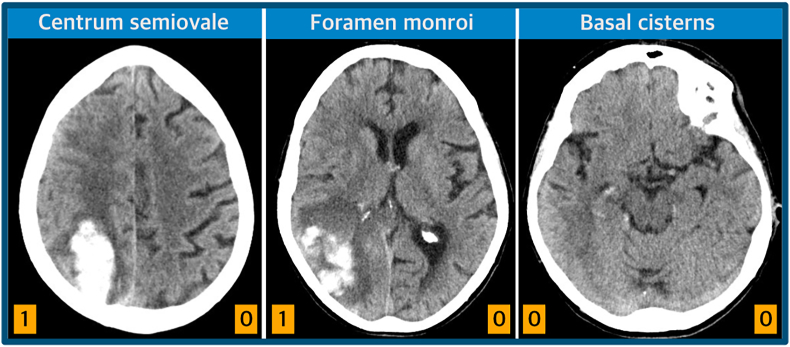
Intracerebral Mass and Brain Edema Score (IMBES). Three defined axial CT slices at the centrum semiovale, interventricular foramen of Monro and basal cisterns were visually inspected by two independent reviewers. Sulcal effacement of the subarachnoid space, involving more than half of the hemisphere, resulted in the assignment of one point. Assessment of the subarachnoid space by sulcal effacement for each hemisphere results in an IMBE-score between 0 and 6 points.
